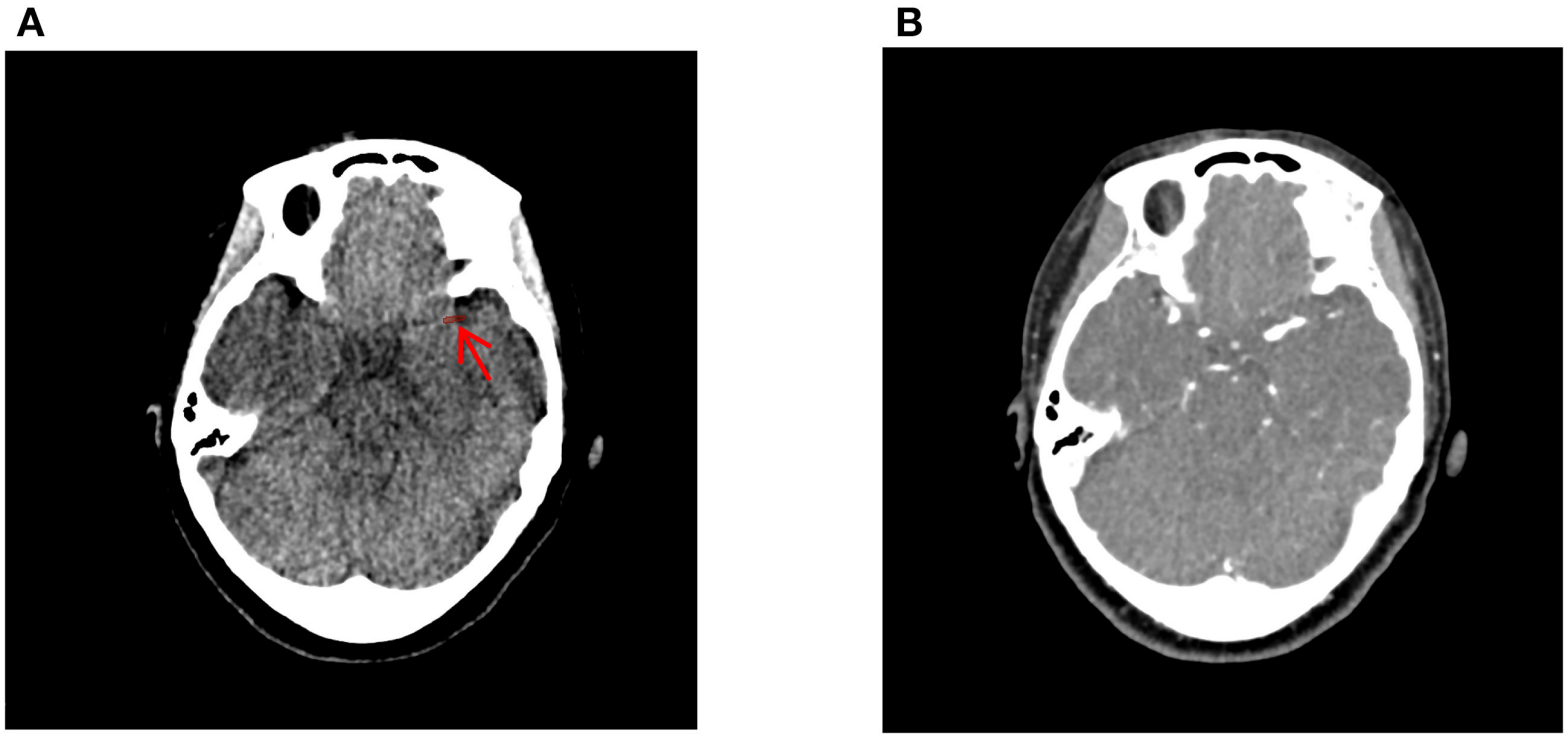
Delineation of the thrombus using the 3D-slicer software. The thrombus on the non-contrast computed tomography (A) was manually segmented using the computed tomography angiography image (B) as guidance. The red arrow indicates the location of the thrombus ROI.
